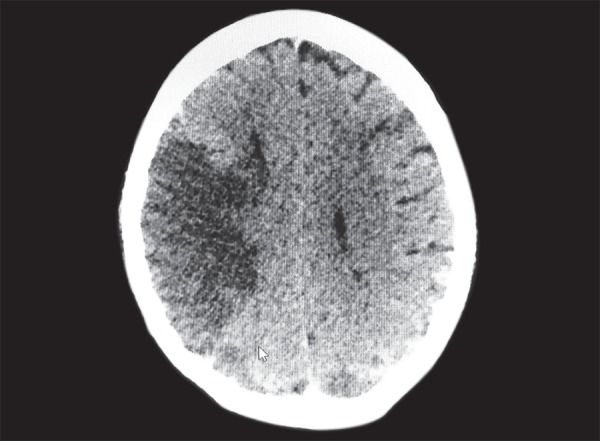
CT brain showing large MCA infarct with multiple small infarcts at other sites
